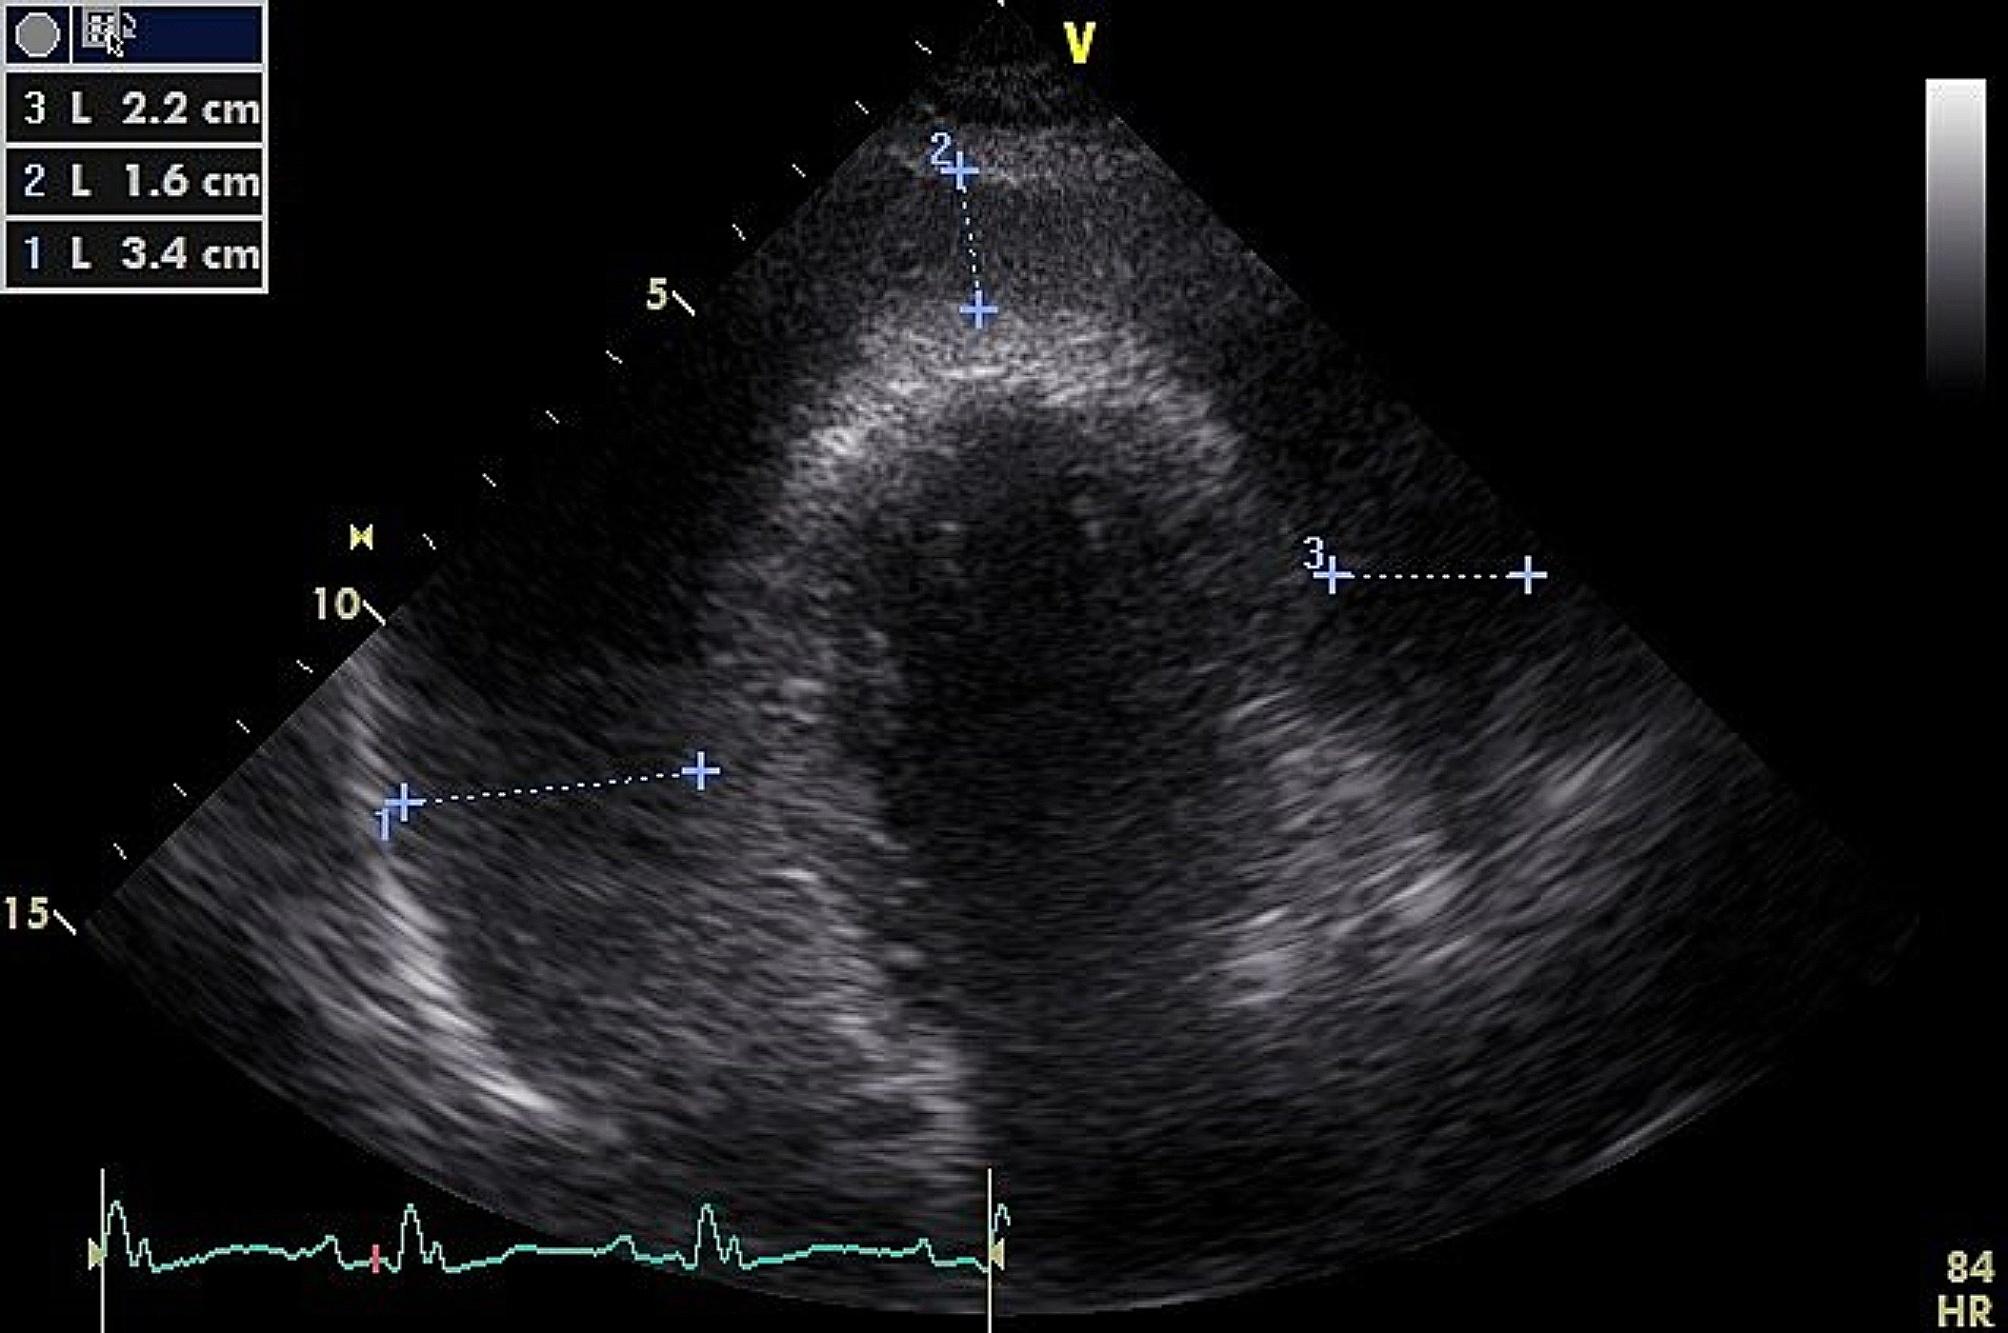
Echocardiogram showing massive pericardial effusion
